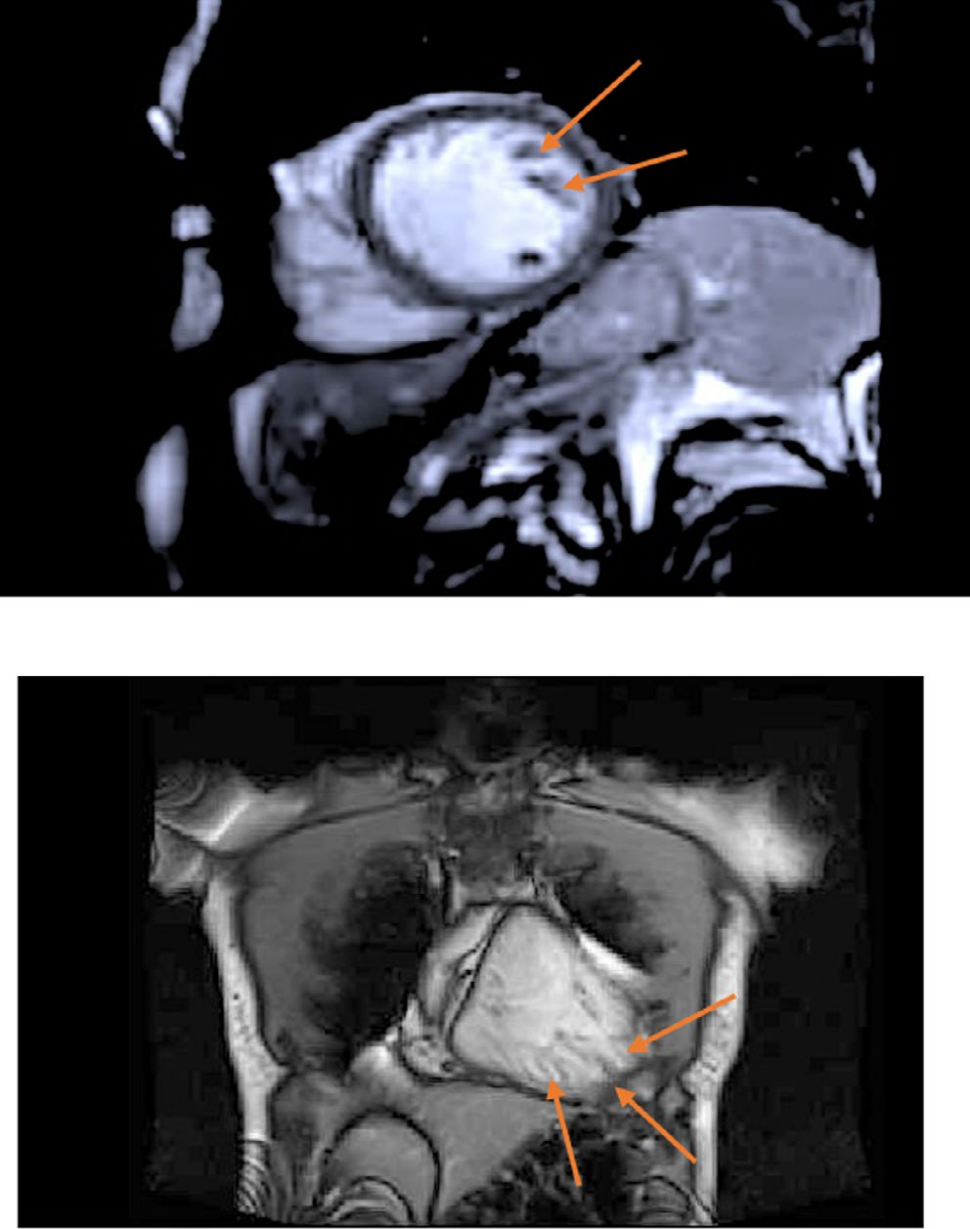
Cardiac MRI images showing trabeculations with deep recesses suggestive of LVNC.
